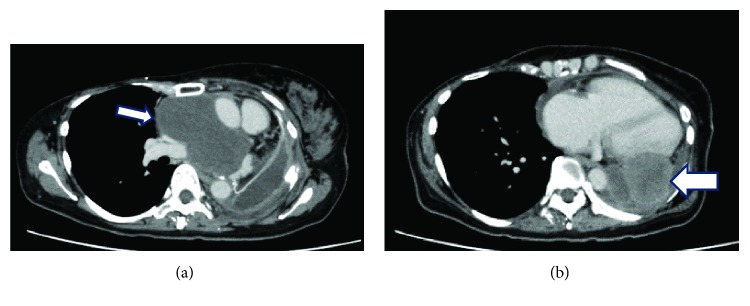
Computed tomography scan acquired 4 months after lung surgery showing (a) a 10 cm mass in the mediastinum (arrow) and (b) a 6 cm mass in the left thoracic cavity (arrow).
